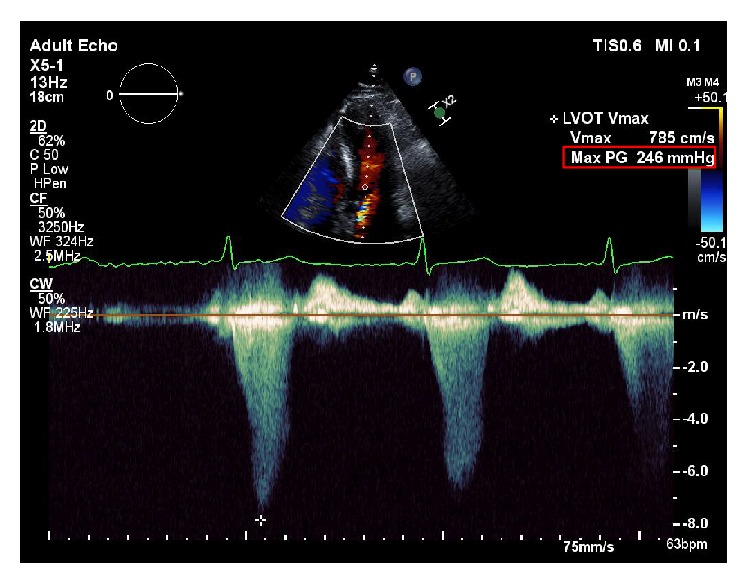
Preoperative Transthoracic Echocardiogram demonstrating left ventricular outflow tract pressure gradient of 246 mmHg with Valsalva.
